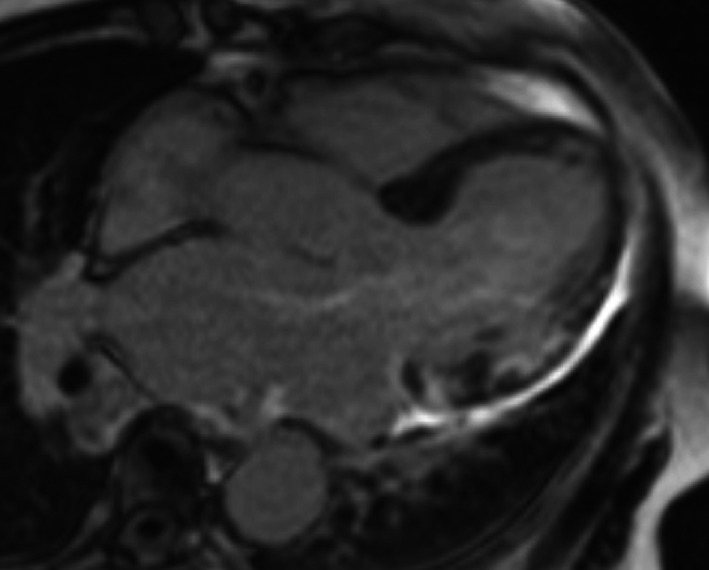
Late gadolinium enhancement (LGE) with longitudinal cardiac magnetic resonance imaging
